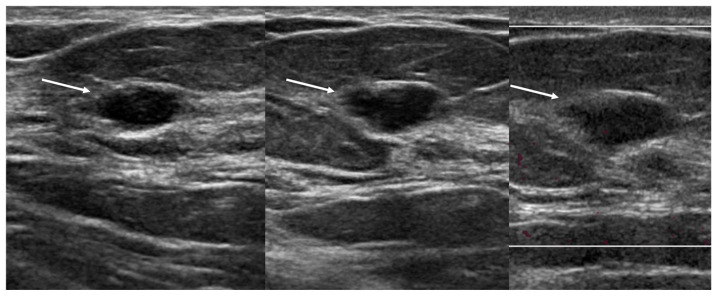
False-negative AI assessment of TNBC. This 48-year-old woman was recalled from screening tomosynthesis (not shown) for a new, indistinctly marginated mass left breast. Targeted transverse (left), sagittal (middle), and sagittal power Doppler (right) US images show an oval 7-mm anechoic mass described as a possible complicated cyst on US review, but clinically assessed as BI-RADS 4B due to mammographic features. In the validation set, the KDS score was 0.41 (a probably benign rating). Core biopsy showed grade 3 TNBC.
